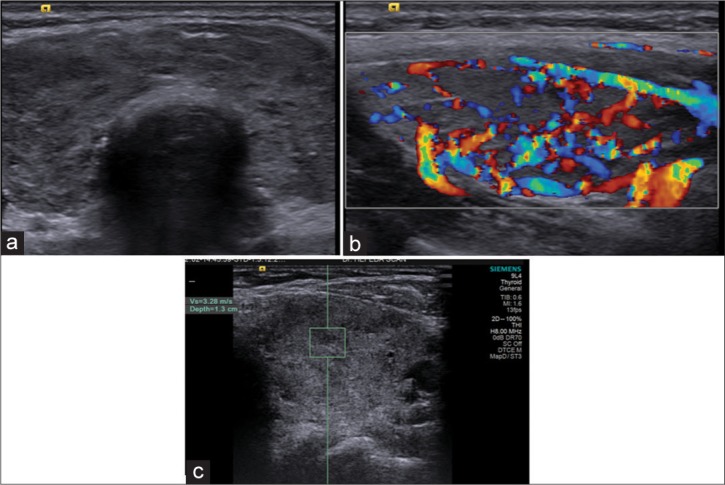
Patient with Hashimoto’s thyroiditis: (a) Grayscale ultrasound showing hypoechoic heterogeneous echopattern with miconodularity. (b) Color Doppler: Hypervascularity with Type II pattern. (c) Shear wave velocity 3.28 m/s (depth 1.3 cm).
